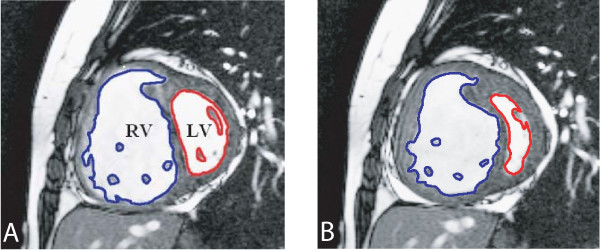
Double-oblique steady-state free precession cine MR images (repetition time ms/echo time ms 3.3/1.65; flip angle 60 deg; section thickness, 6 mm; matrix, 256 × 150). End-diastolic (a) and end-systolic (b) cardiac short axis slice in PAH patient. The endocardial boundaries of the right ventricle (RV) and left ventricle (LV) are traced for calculation of end-diastolic volume (EDV) and end-systolic volume (ESV). EDV and ESV were calculated by summation of the product (area × slice distance) for all slices. SV is then given by SV = EDV-ESV for RV and LV.
